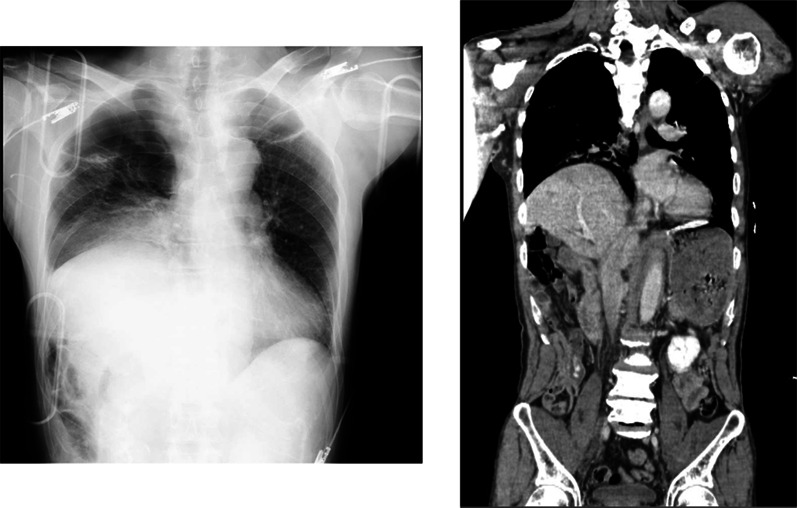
Chest X-ray and CT scan findings on the day of admission. The enhanced CT scan and chest X-ray shows right diaphragm elevation and pulmonary contusion
